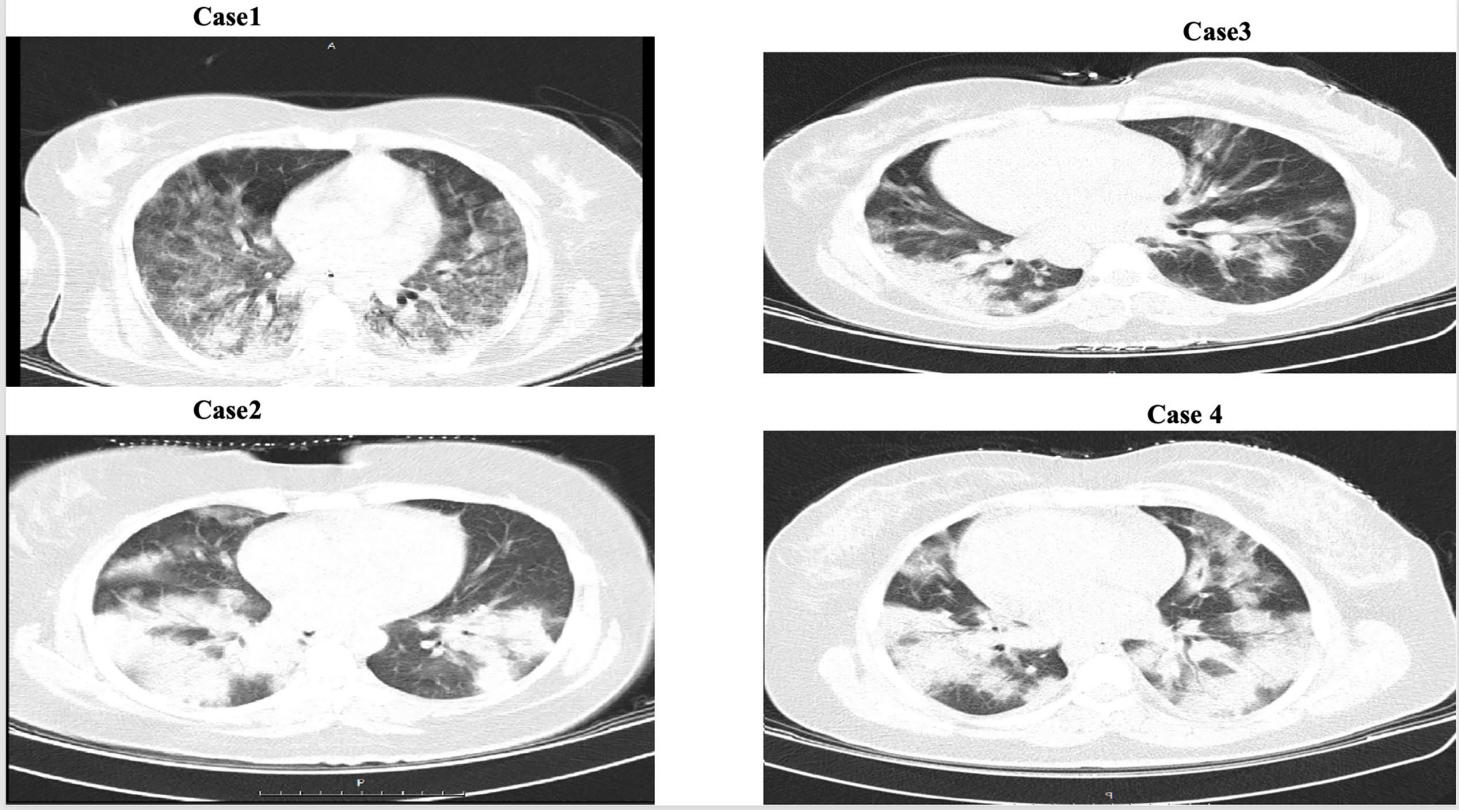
CT scan of the lung patients.
